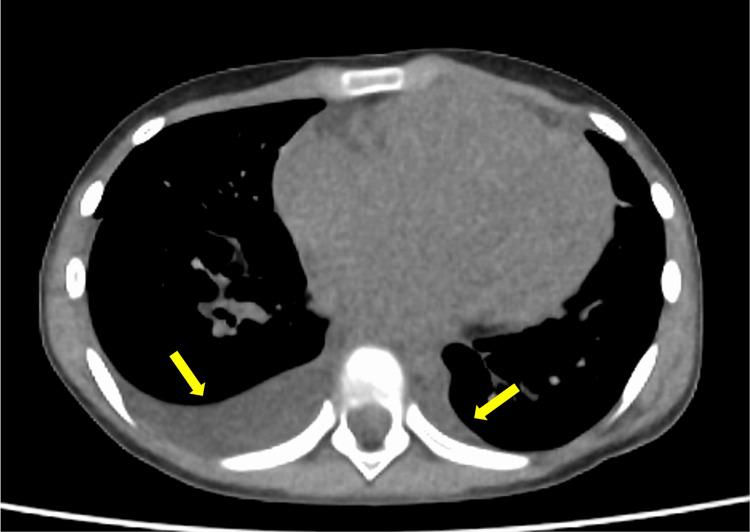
Axial PET-CT image showing pleural effusion (arrows) and cardiomegaly. CT images were acquired with a slice thickness of 4.0 mm. PET imaging was performed 60 minutes after intravenous administration of fluorine-18 fluorodeoxyglucose at a dose of 112 MBq.
